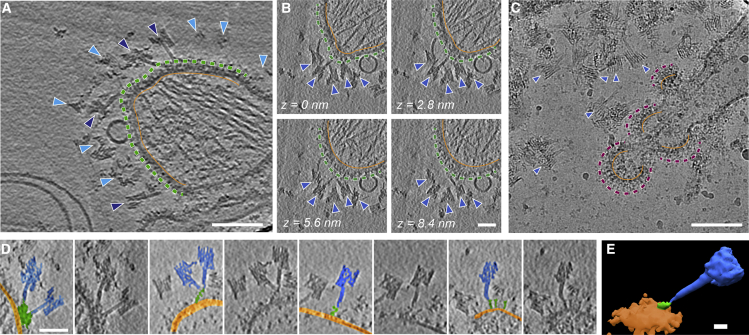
SPOTs binding to sfGFP-gB vesicles observed by cryoET Computed tomogram slices in all panels are 2.8 nm thick. (A) Computed slice from a high-magnification tomogram (1.34 Å/pix) showing SPOTs binding selectively to sfGFP-gB vesicles. The distal edge of sfGFP-gB is indicated by a green dashed line; an orange line is drawn just below the membrane surface. SPOTs are indicated with dark blue arrow heads if their posts are visible in the tomogram slice and light blue if they are situated across multiple slices. Full views of all slices from the tomogram are shown in Figure S4. Average defocus across the tilt series was ∼−3.8 μm. Scale bar, 100 nm. (B) Sequential computed tomogram slices showing SPOTs bound to sfGFP-gB. Structures are labeled as in (A). Scale bar, 50 nm. (C) SPOTs are not observed binding to the vesicles in a projection image when gB is tagged with mCherry instead of sfGFP. Magenta dashed lines indicate the distal protein face, and orange lines indicate the inside of the membrane. mCherry belongs to a different family of fluorescent proteins than sfGFP. Scale bar, 100 nm. (D) Gallery of images of individual SPOTs extracted from tomograms of labeled sfGFP-gB vesicles. Each image is shown twice, with and without annotation on the left and right, respectively. SPOTs are highlighted in blue, sfGFP-gB in green, and the membrane in orange. It is often possible to follow the SPOT posts directly to the recognized protein density on the vesicle surfaces. Scale bar, 50 nm. (E) A sub-volume average of SPOTs (blue) bound to sfGFP-gB (green) in vesicle membrane (orange) with the orientations determined by the positions of the posts of the SPOTs as initial alignment markers. Scale bar, 10 nm.
